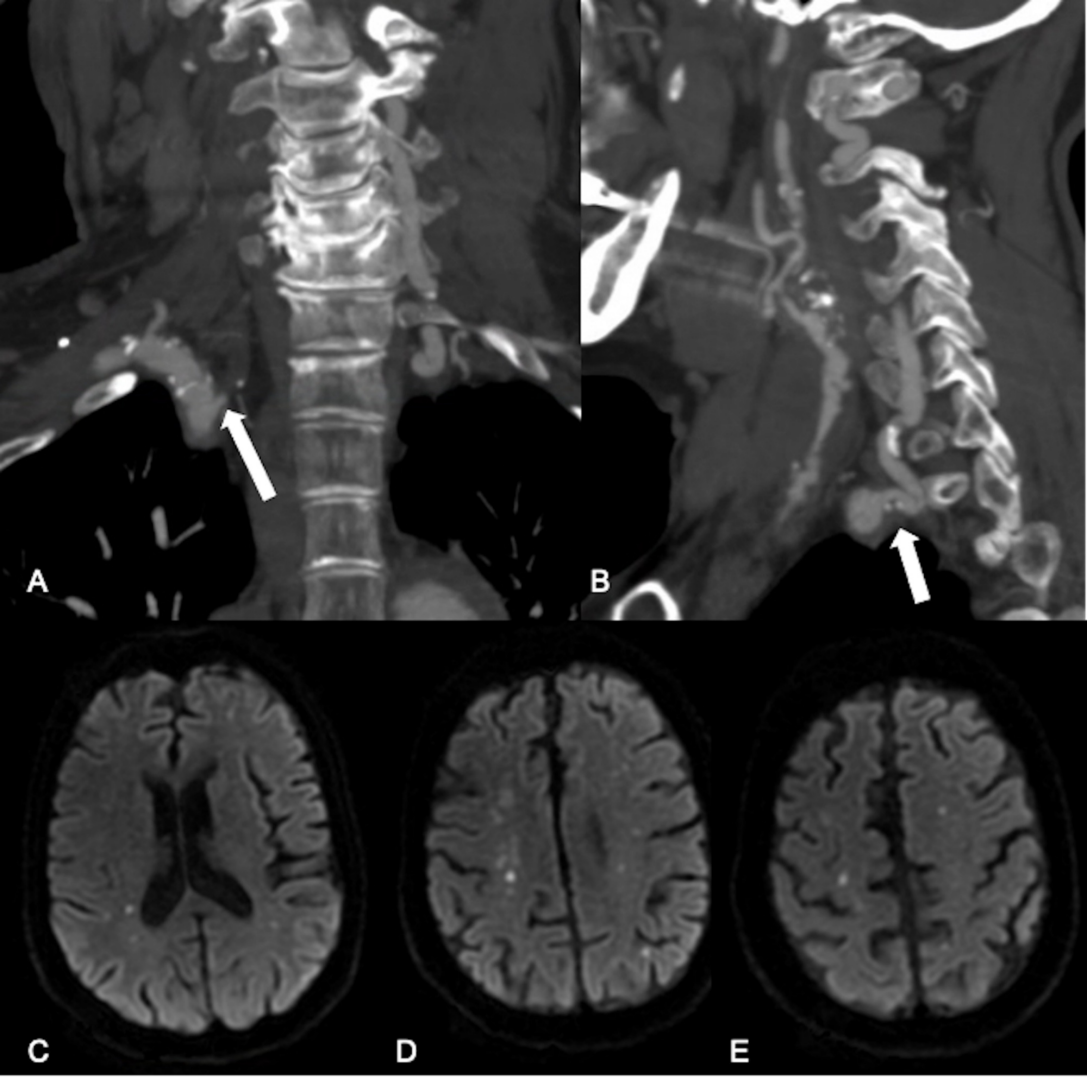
Bilateral vertebral artery disease and bihemispheric anterior circulation infarcts A, B - CT angiography coronal and sagittal reconstructions: occlusion of the right vertebral artery at its origin with visualization of a vessel stump (A, arrow). Moderate stenosis at the origin of the left vertebral artery due to mixed calcified and non-calcified plaque (B, arrow). C, D, E - MRI DWI axial slices: magnetic resonance imaging of the brain reveals multiple small infarcts in both cerebral hemispheres (C-E).
